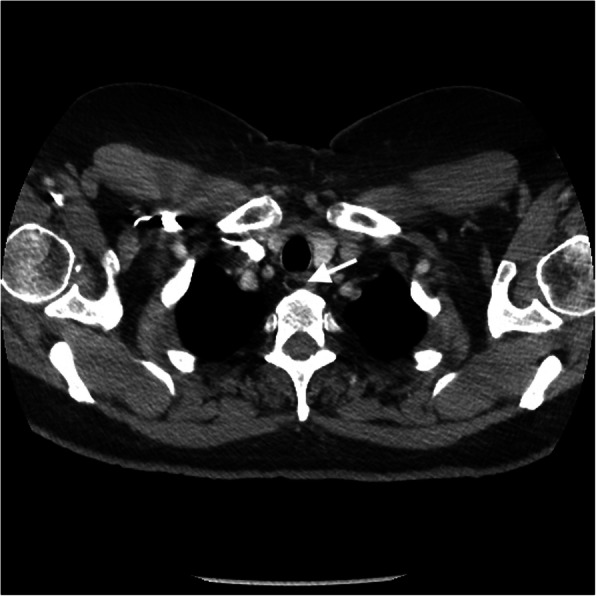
Fibrovascular polyp. Axial CT image of the superior thorax demonstrates a fat attenuation lesion in the right lateral esophageal wall in the upper esophagus (arrow) consistent with predominant adipose tissue
